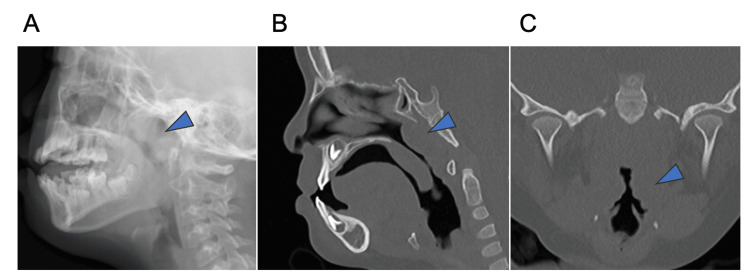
Physical examination and findings of patient 5 X-ray (A) and CT-scan (B: sagittal section, C: coronal section) show adenoid and tonsil hypertrophy (blue triangle).
